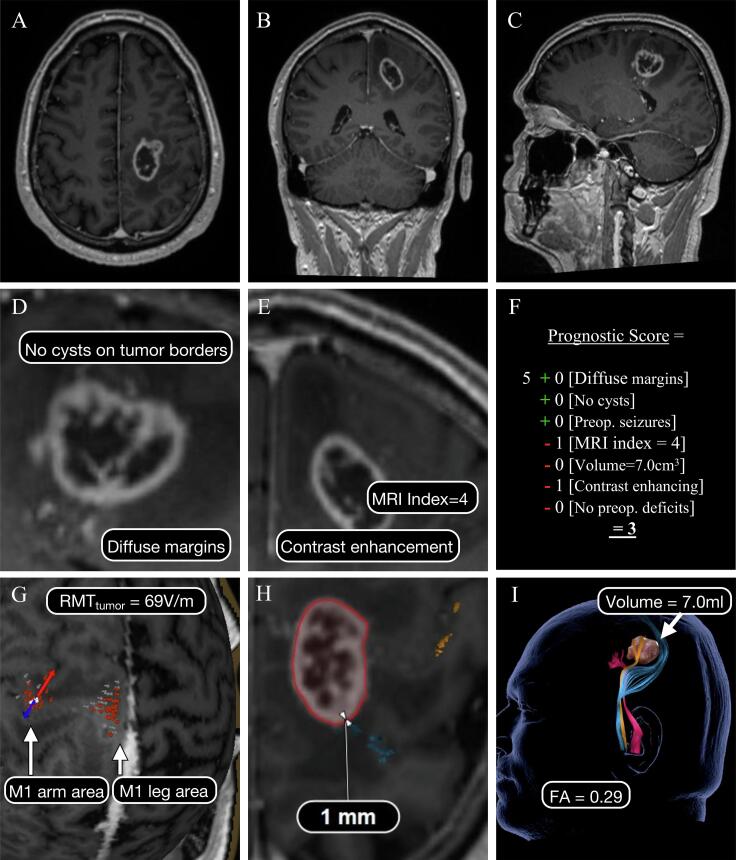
Application of the PrS and nTMS model to an exemplary case. MRI scan (T1 with contrast agent) show a left central tumor (A-C) of a 62-year-old man. Diffuse margins of the contrast-enhancing tumor, an MRI index of 4 and no peritumoral cysts (D, E) equate to a a PrS of 3, meaning 8% chance for GTR and 50% risk of neurologic deterioration (F). The tumor is located subcortically under the motor cortex. Thus, the nTMS mapping did not demonstrate a tumorous infiltration of the motor cortex (G) but an impaired neurophysiological excitability (RMT_tumor_ = 69 V/m; RMT_ratio_ = 82%). The colored spots mark stimulation sites where motor-evoked potentials with different amplitudes were elicited. These functional areas were used as region of interests for tractography to somatotopically visualize the CST for the lower extremity (blue), upper extremity (yellow), and corticonuclear tracts (pink) (H, I). Due to compromised white matter integrity (FA = 0.29) and TTD of 1 mm, there is a 30.3% risk for transient and 15.6% risk for permanent motor deterioration. A GTR could be achieved but the patient suffered a 2/5 hemiparesis on discharge which recovered to BMRC grade 4/5 after 3 months.
